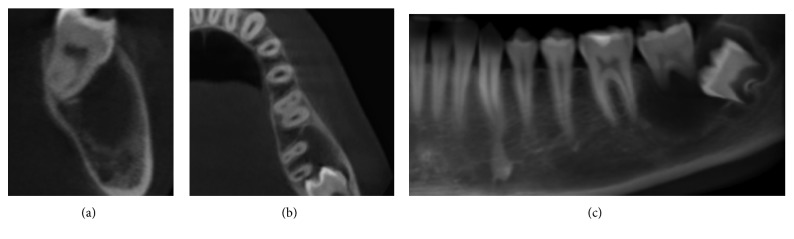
Preoperative radiographic examination: CBCT panoramic reconstruction, showing low-density image extending from the distal root of 36 (a), englobing the radicular part of 37 and the crown of 38 at baseline; axial cut showing extension of the lesion in buccolingual view, with thinning of the buccal cortical without interruption and displacement of 37 in the lingual direction; cross-sectional view at the level of 37 (b), showing clearly the displacement in lingual direction (c).
